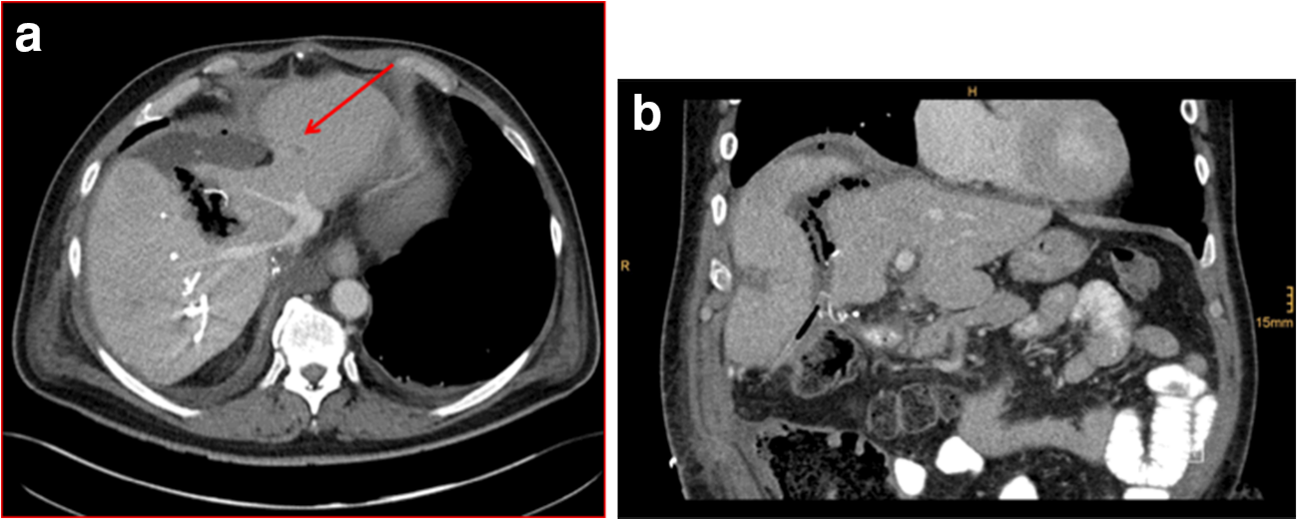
a Contrast-enhanced CT scan (venous phase) 7 days after ALPSS step 1 (17.09.2019) in transverse plane showing a small suspicious lesion in segment II (red arrow) (a). b Coronal view (b)
